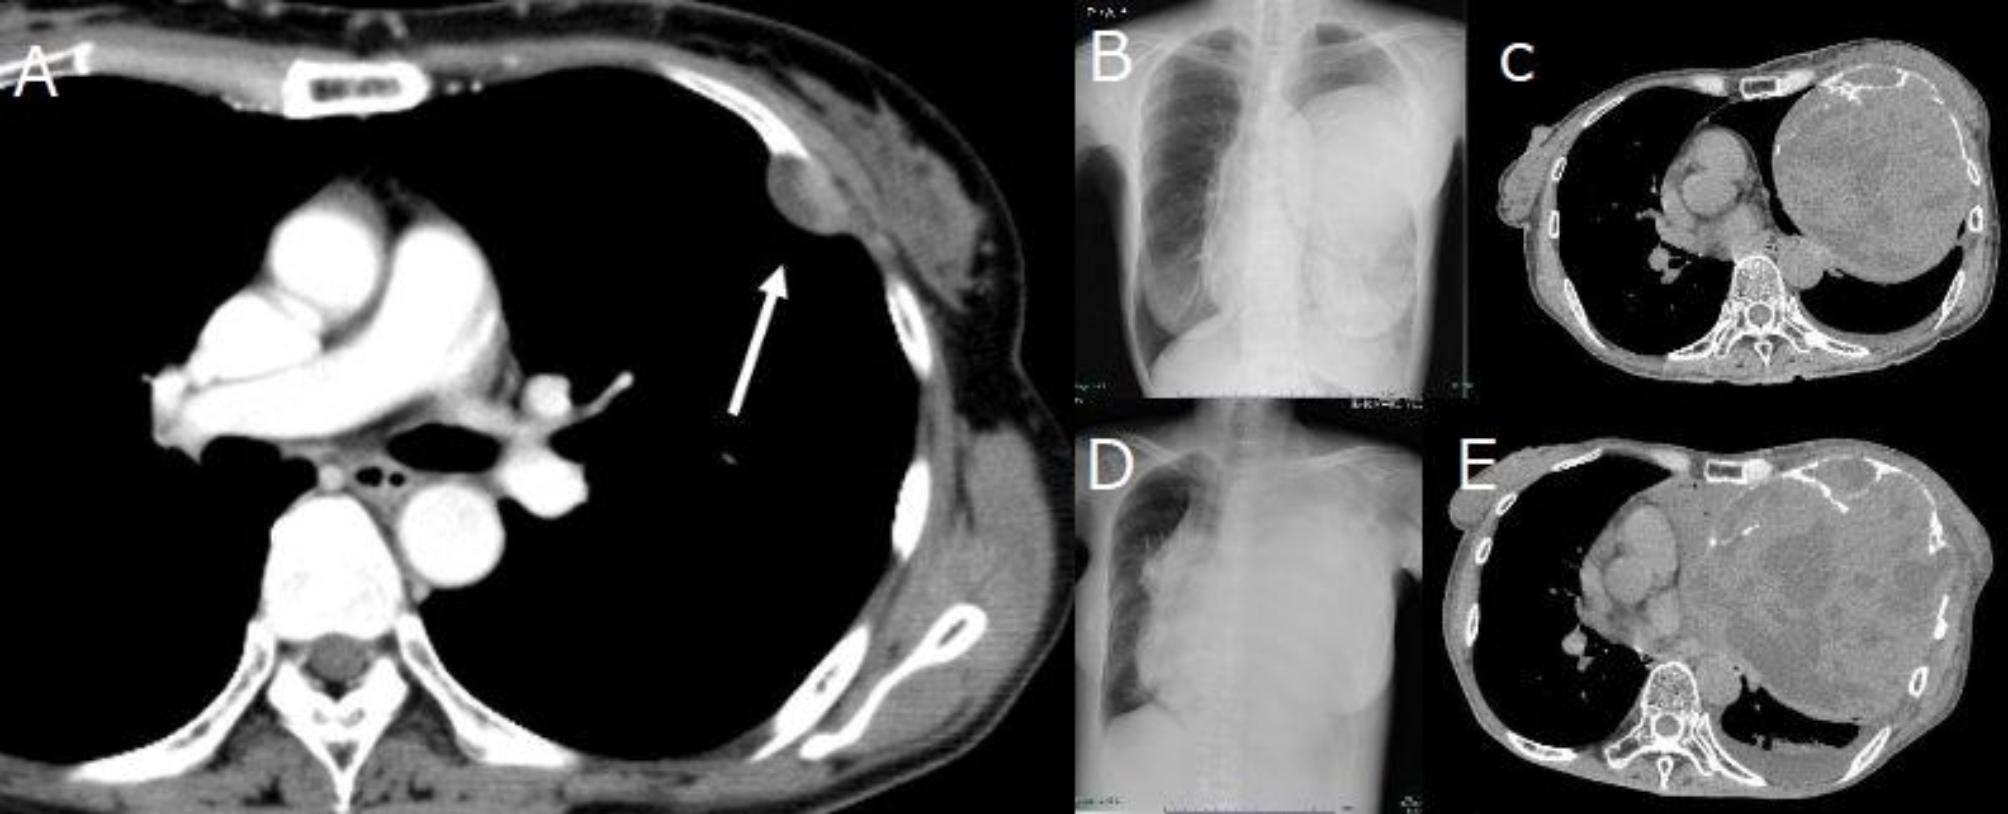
(A) A CT taken 10 years previously shows a small nodule (3.0 × 1.5 cm) in the left third intercostal space(arrow). A chest radiograph (B) and CT scan (C) at the time of referral to our hospital, show a large left chest wall mass of 130-mm in size. Tumor has a fluid component and calcification inside. The ribs are also partially destroyed. A chest radiograph (D) and CT scan (E) show further tumor enlargement, a completely atelectatic left lung, and compression of the mediastinum
